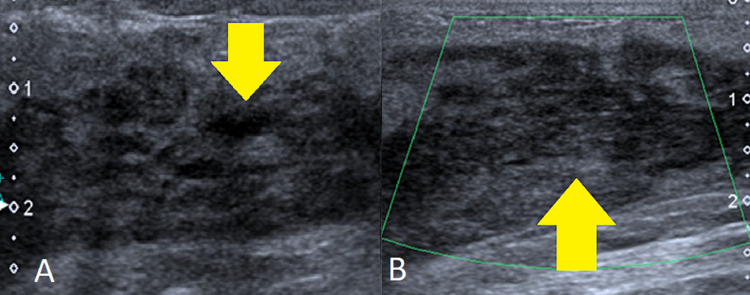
Ultrasound of the right breast Grayscale (A) and color Doppler (B) ultrasound images showing heterogenous infiltrative mass with internal cystic changes diffusely involving the outer quadrant and central part of the right breast (yellow arrows). No vascularity is seen on color Doppler images.
